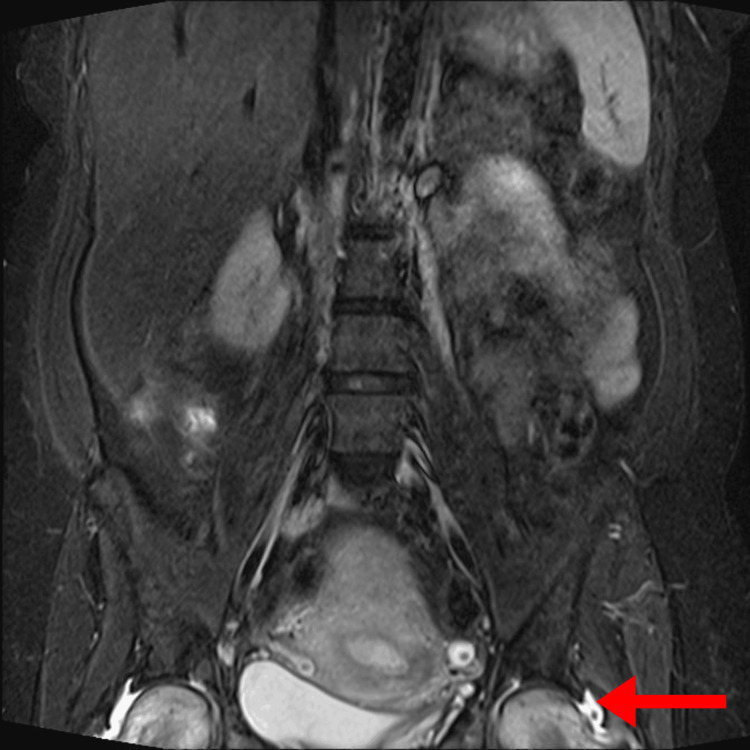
MRI of the lumbosacral spine showing bilateral femoral head STIR hyperintensities MRI, magnetic resonance imaging; STIR, short tau inversion recovery.
